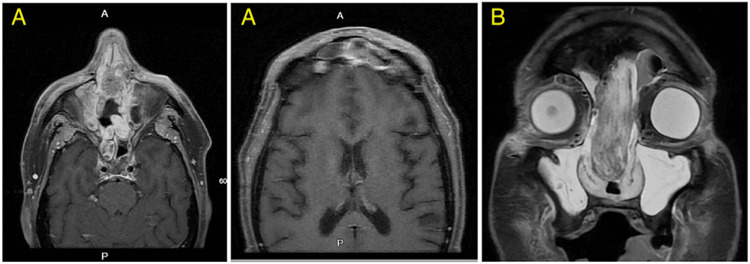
Three-month post-treatment MR images including axial (A) and coronal (B) T1 post-contrast sequences and coronal STIR sequence STIR: short tau inversion recovery
